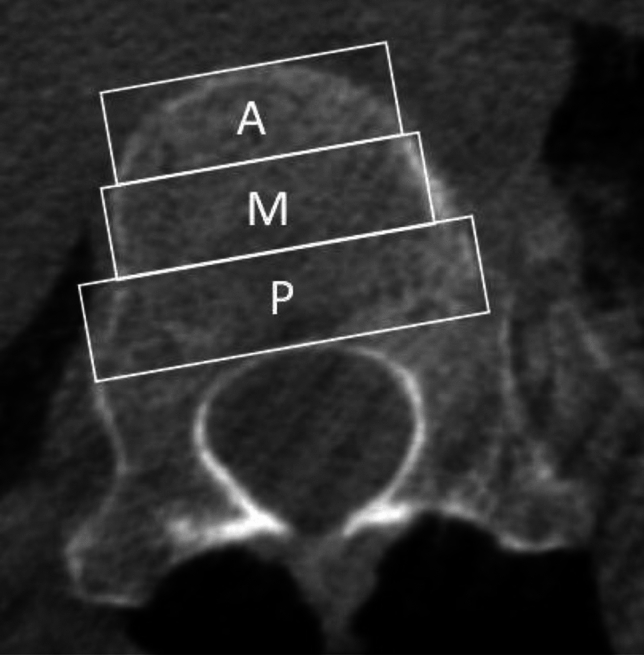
Division of vertebral bodies into anterior (A), middle (M), and posterior (P) thirds on axial CT images to document screw-starting and -ending points
